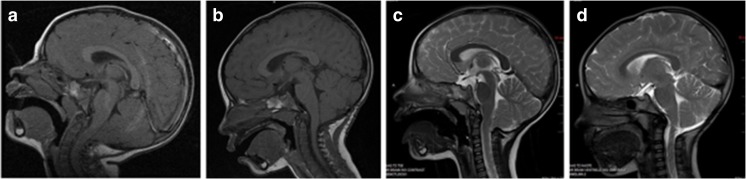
A 30-month-old girl (Case 16) who presented with increasing irritability, difficulty to swallow, and tendency to fall. In past several days, the patient woke up screaming. Midsagittal T1-weighted MR showed a Chiari I malformation with tight posterior fossa with the tonsils below the C1 level (A). A posterior decompression with dural patch grafting was done. Postoperative MR done 3 months later showed increased subarachnoid space but the position of tonsillar tips remained unchanged (B). Her symptoms resolved shortly after the surgery. However, 9 month later, she started to experience increasing headaches. Midsagittal fast T2-weighted MR done 13 months postoperatively showed the tonsils descending at the level of the previous C1 and C2 junction with epidural tissue growth causing constriction at that level (C). At reoperation, a thick fibrous band at C1 level was removed. An expansion of thecal sac was observed without opening the dura. MR done 2 years after the second surgery showed excellent decompression and she was asymptomatic (D).
